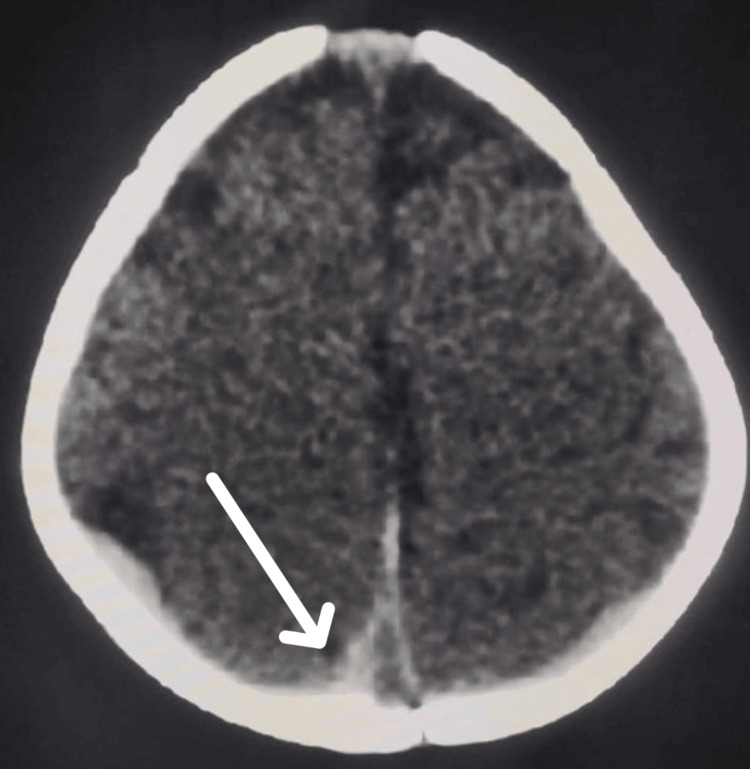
MDCT brain showing a thin rim of subdural hemorrhage along the bilateral parietal parafalcine and parieto-occipital regions MDCT: multidetector computed tomography
